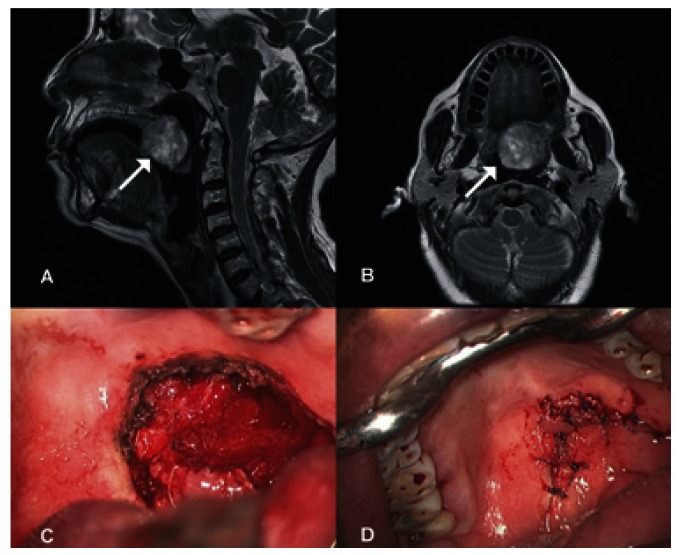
A) Sagittal MRI showing lesion in the soft palate without compromise of the nasal cavity. B) Axial MRI with lesion found to the left of the midline, dependent on the soft palate. C) Photo after resection showing the defect in the soft palate. D) Photograph showing primary closure in the immediate postoperative period.
